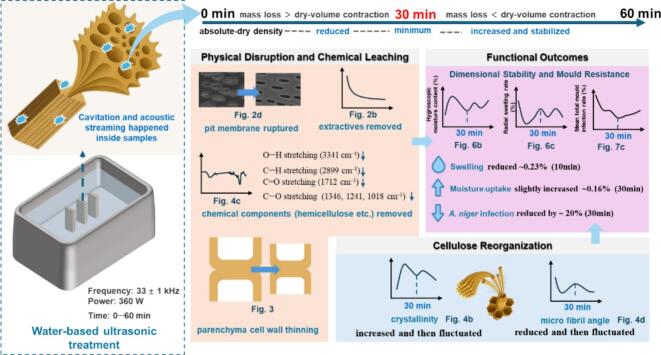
Mechanistic pathway from water-based ultrasonication to improved dimensional stability and antifungal performance in moso bamboo.
